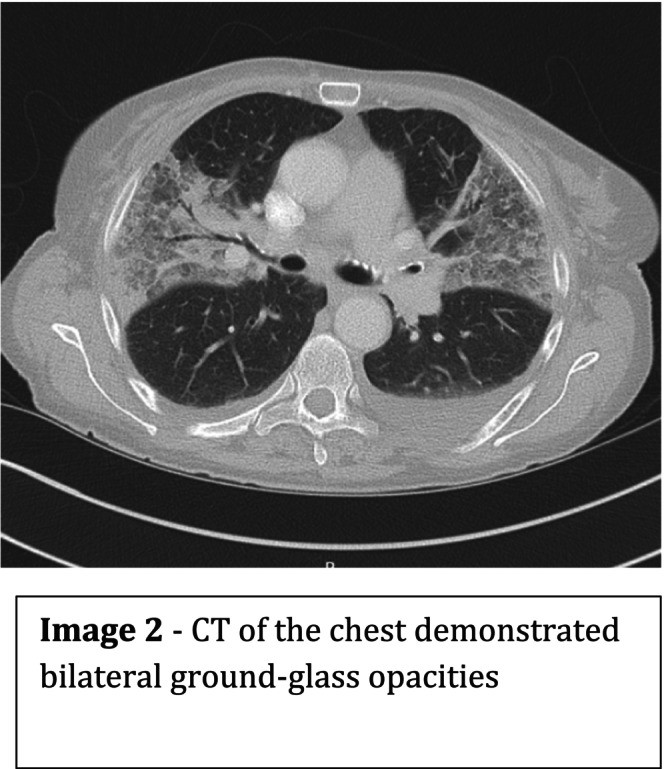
CT of the chest demonstrated bilateral ground‐glass opacities.
